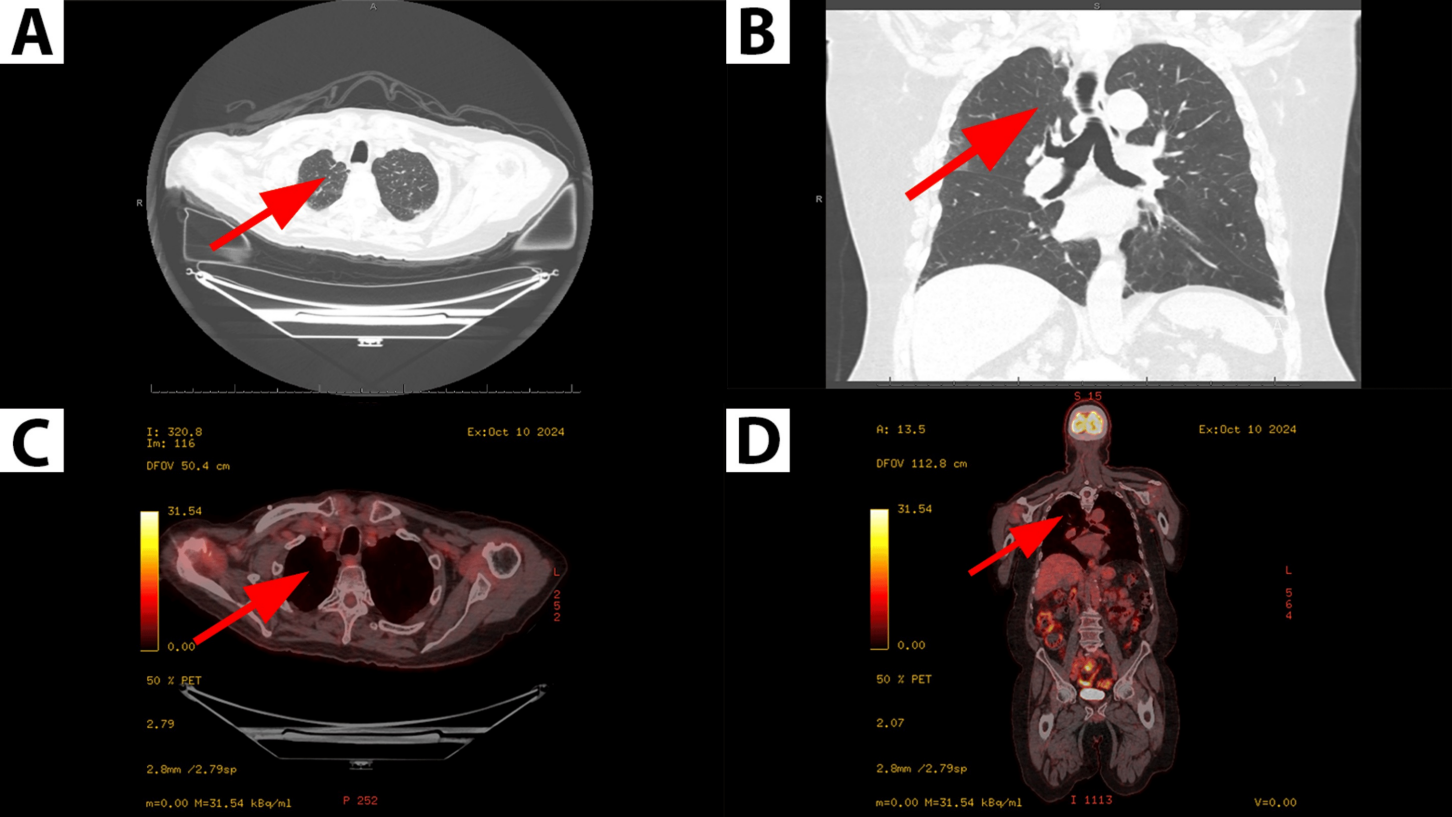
Three-year follow-up axial and coronal images of chest CT and PET-CT. A and B: Axial and coronal views of chest CT showing reduced size of right apical mass (red arrow) and less disease burden. C and D: Axial and coronal views of PET-CT showing reduced hypermetabolic activity (red arrow).
